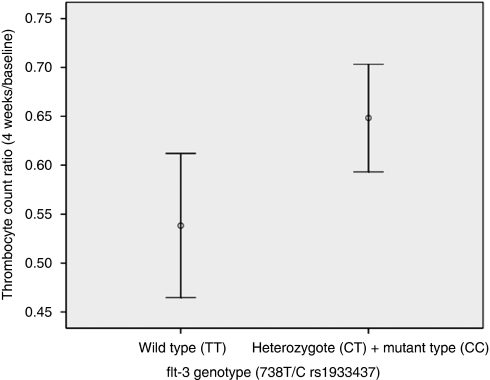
Trombocyte counts decrease after sunitinib start in flt3 738 TT *vs* CT and CC carriers.
